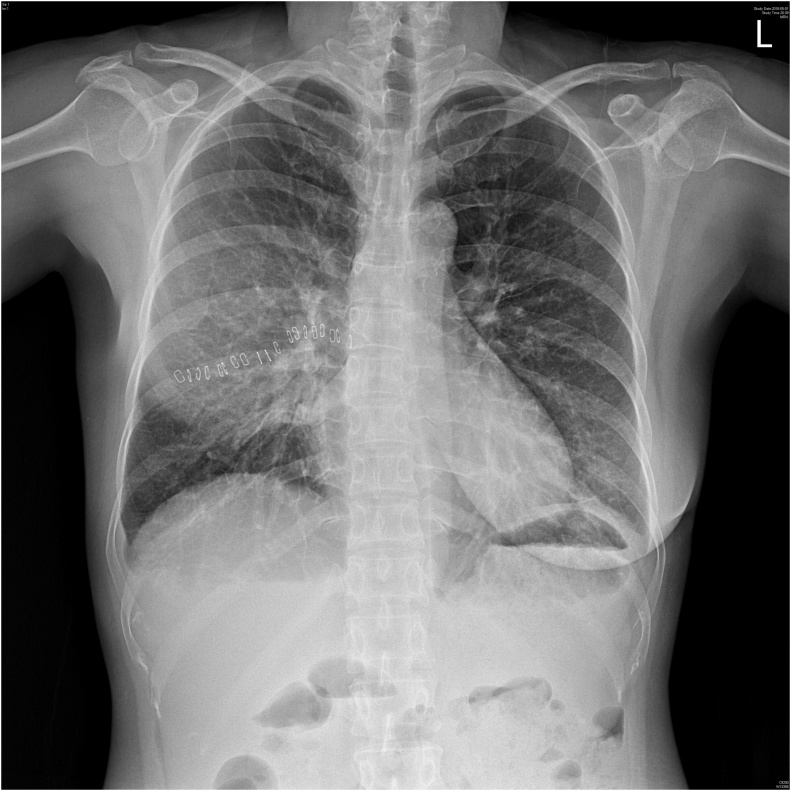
Chest x-ray on post-operative Day 20. Soft haziness in the right middle lung field and costophrenic angle blunting with subsegmental atelectasis in left lower lung field are observed.
